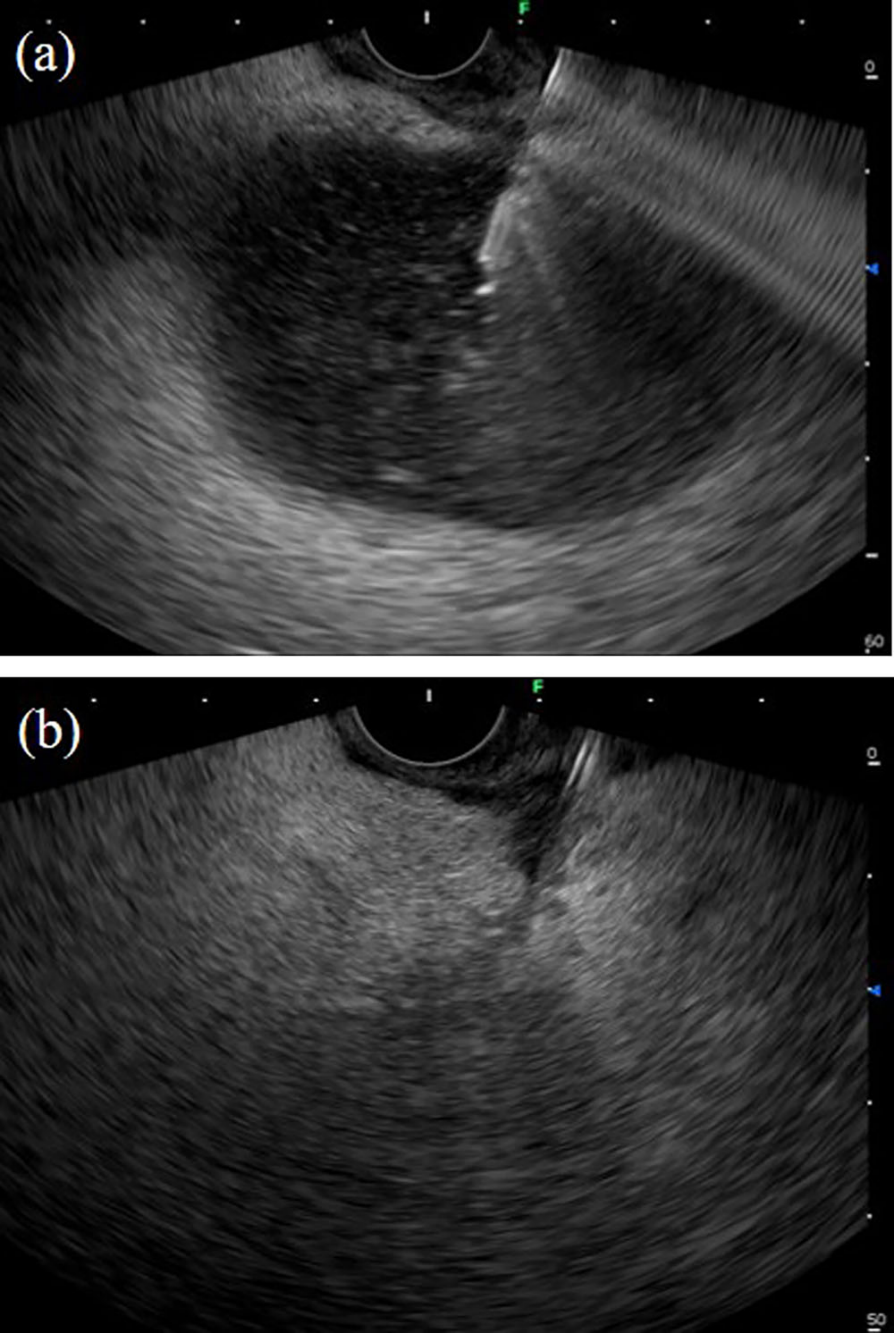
(a) The pancreatic fluid collection was identified by ultrasound. A 19‐gauge fine needle aspiration needle was used to puncture through the gastric wall into the pancreatic fluid collection. (b) After insertion of a 19‐gauge needle into the pancreatic fluid collection under endoscopic ultrasound guidance, the fluid within was aspirated.
